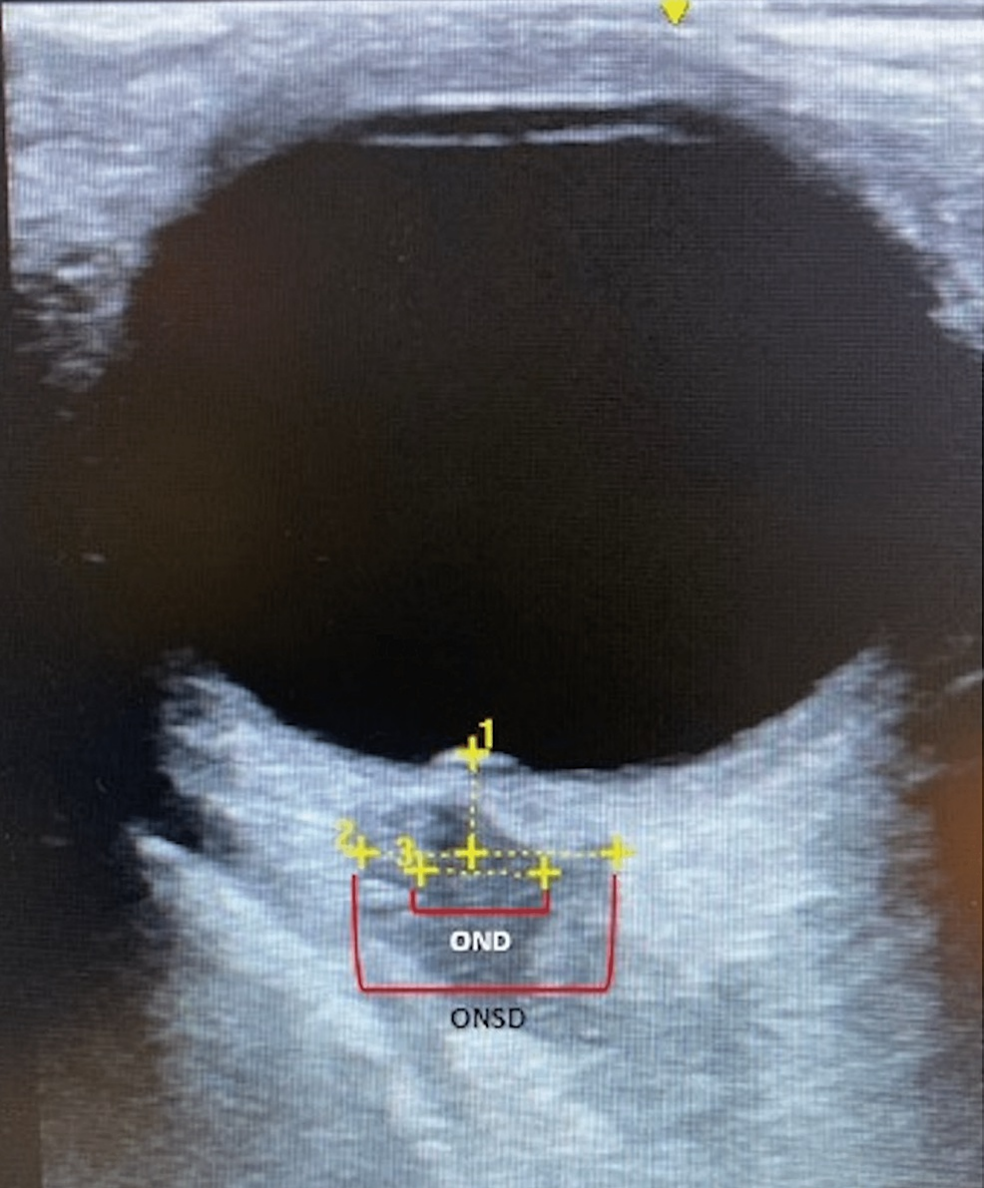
Right ocular ultrasonography in transverse plane. Papilla protrusion is shown which is suggestive of papilledema indicated by #1. A widened optic nerve sheath diameter (ONSD) of 7.6 mm is indicated by #2 and #3 shows the optic nerve diameter (OND). Both findings are suggestive of elevated intracranial pressure. Not pictured were the results of the ultrasonography of the left eye but were comparable in terms of widened optic nerve sheath diameter and papilledema.
